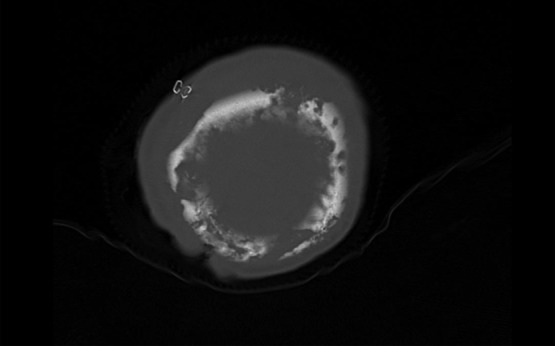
Computed tomographic image demonstrating full-thickness osteomyelitis of the cranium.
